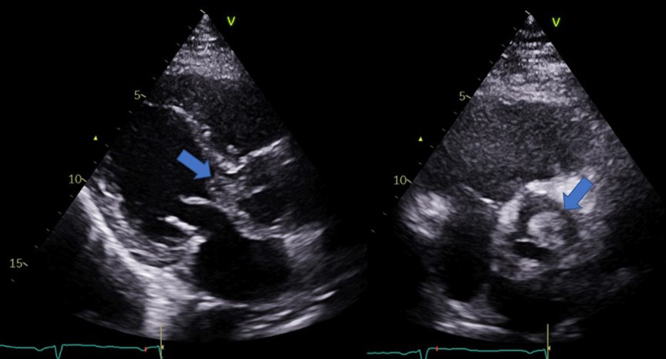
Echocardiography showing a mass (i.e., Fungal ball) attached to the cusps of the aortic valve (blue arrows). (For interpretation of the references to color in this figure legend, the reader is referred to the web version of this article.)
